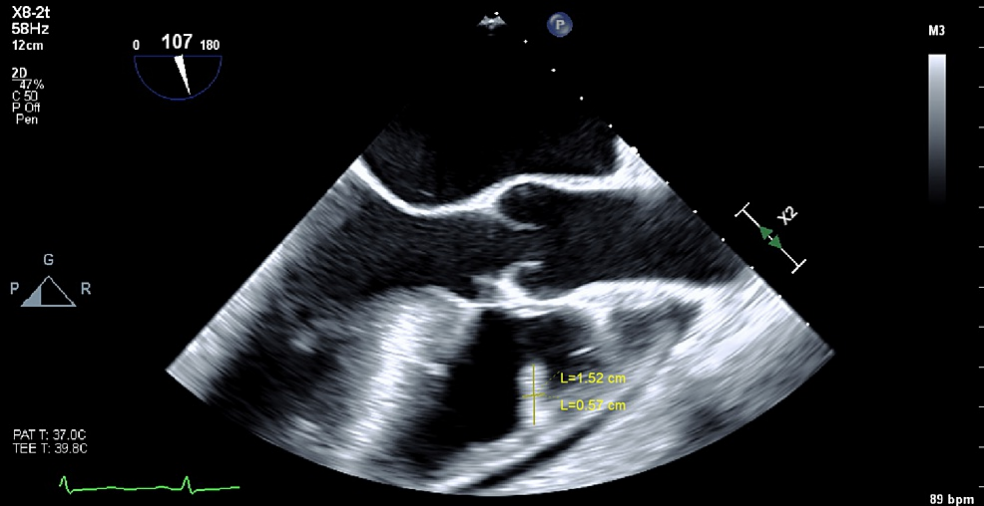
Transesophageal echocardiography (TEE) image showing vegetation (1.52 cm x 0.57 cm) suggestive of a valvular disease
